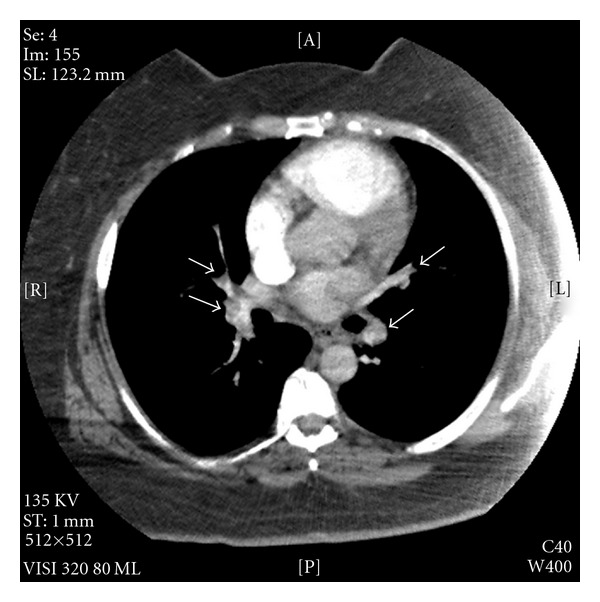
CT angiogram of the chest showing bilateral pulmonary emboli (arrows).
